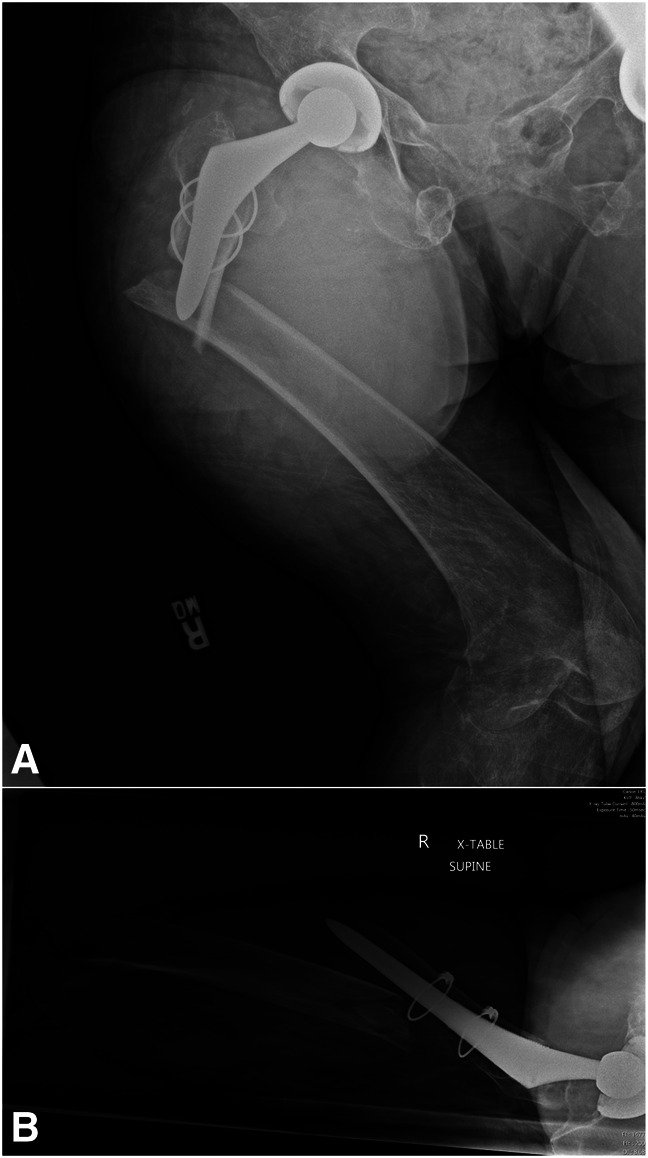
A, AP and (B) lateral radiographs of the patient's right hip immediately upon presenting to the emergency department demonstrate a displaced and comminuted periprosthetic spiral fracture of the proximal femur. The femoral implant appears to remain well-fixed.
